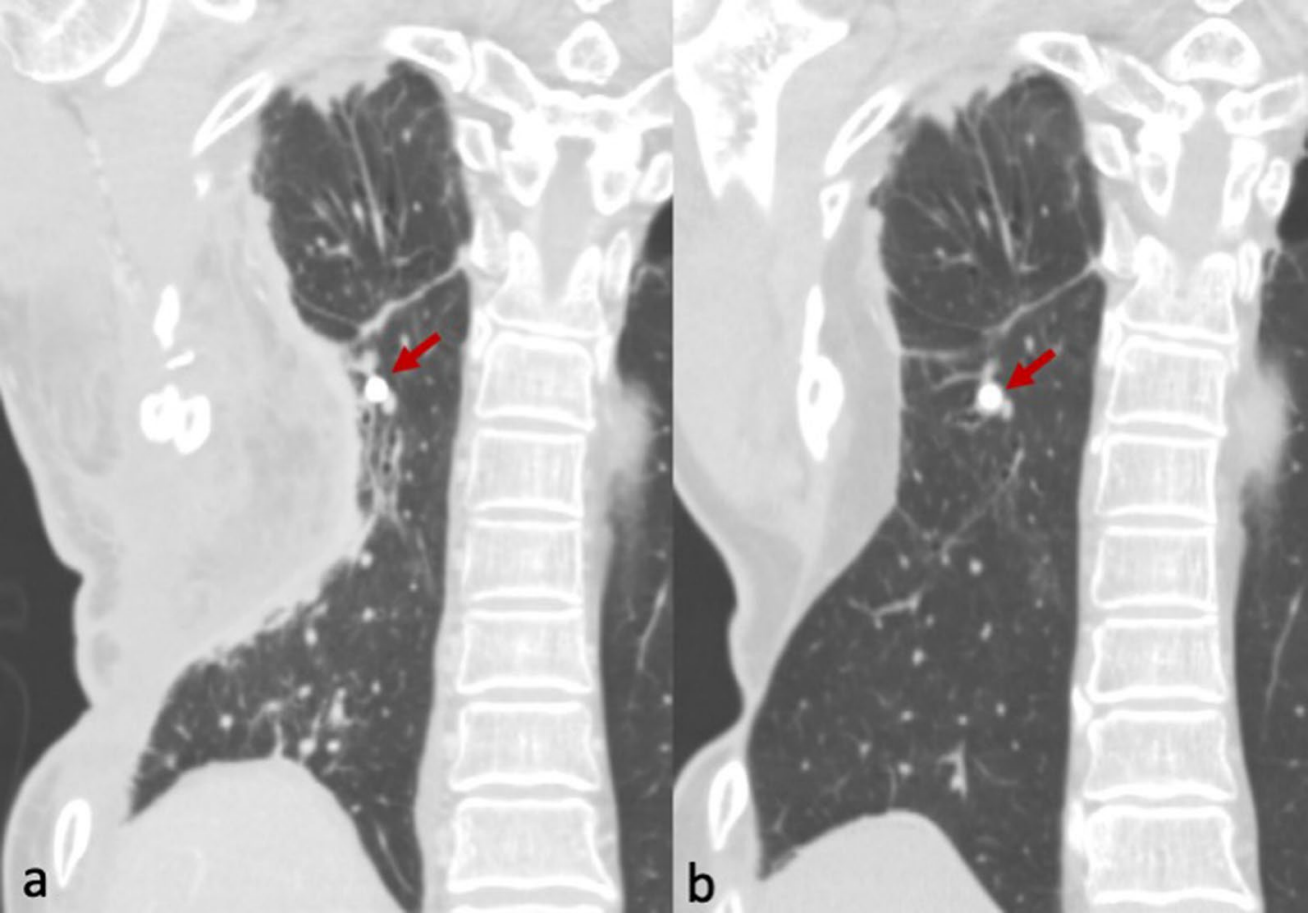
CT images: Immediate post-window closing surgery (a) and six years follow-up (b). The lung demonstrates good expansion, and the EWS remains preserved without any complications (arrows)
